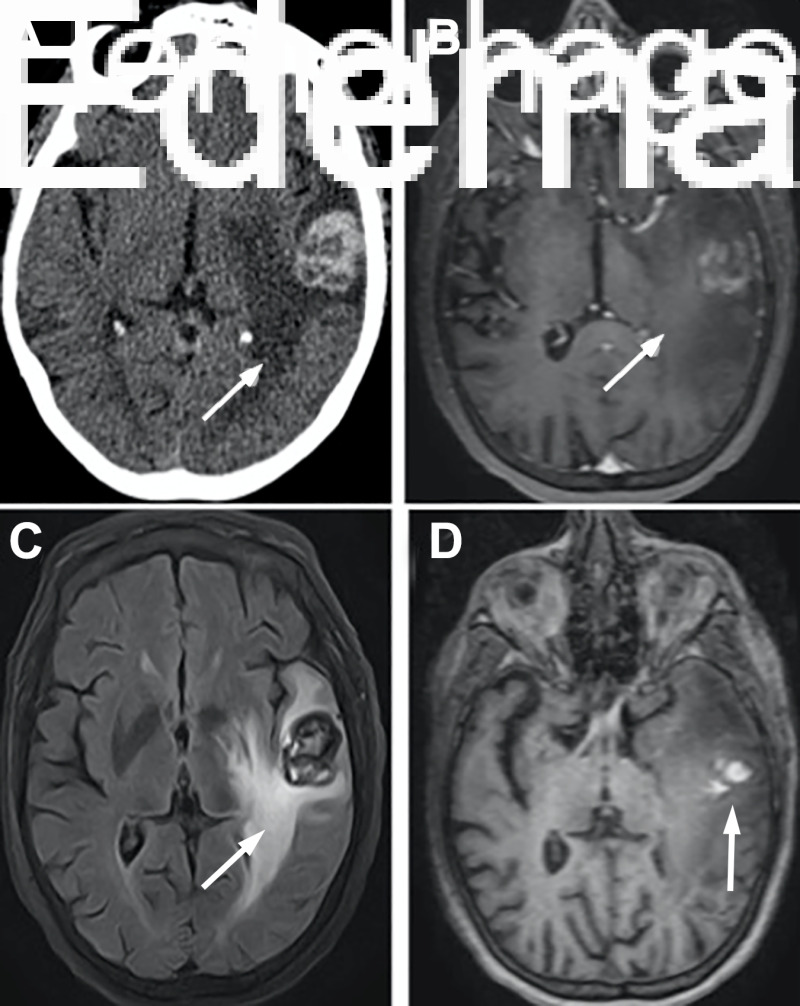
Head computed tomography scan and magnetic resonance imaging (MRI) scans (A) Non-contrast head computed tomography scan, (B) T1-weighted postcontrast enhanced magnetic resonance imaging (MRI), (C) T2 fluid-attenuated inversion recovery MRI demonstrating significant edema (arrows), and (D) T1-weighted precontrast MRI revealing 2.9-cm hyperdensity representing hemorrhagic mass (arrow) in the left temporal lobe found seven years after stereotactic radiosurgery.
